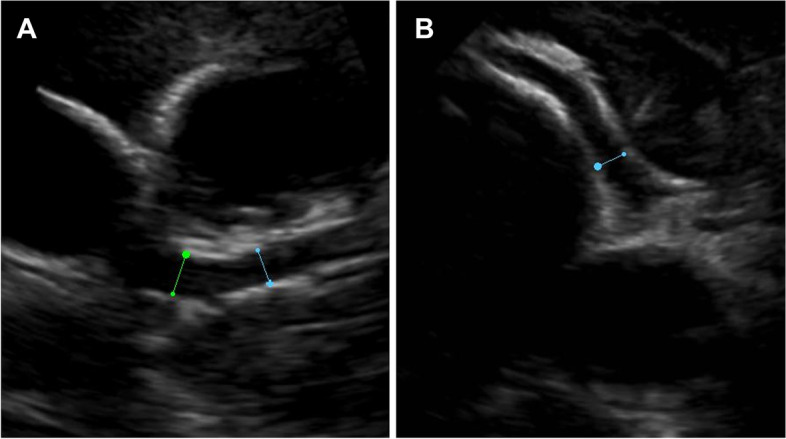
A Echocardiogram images at 2 months of age showing dilated coronary arteries—left main coronary artery and proximal left anterior descending and B right anterior descending
